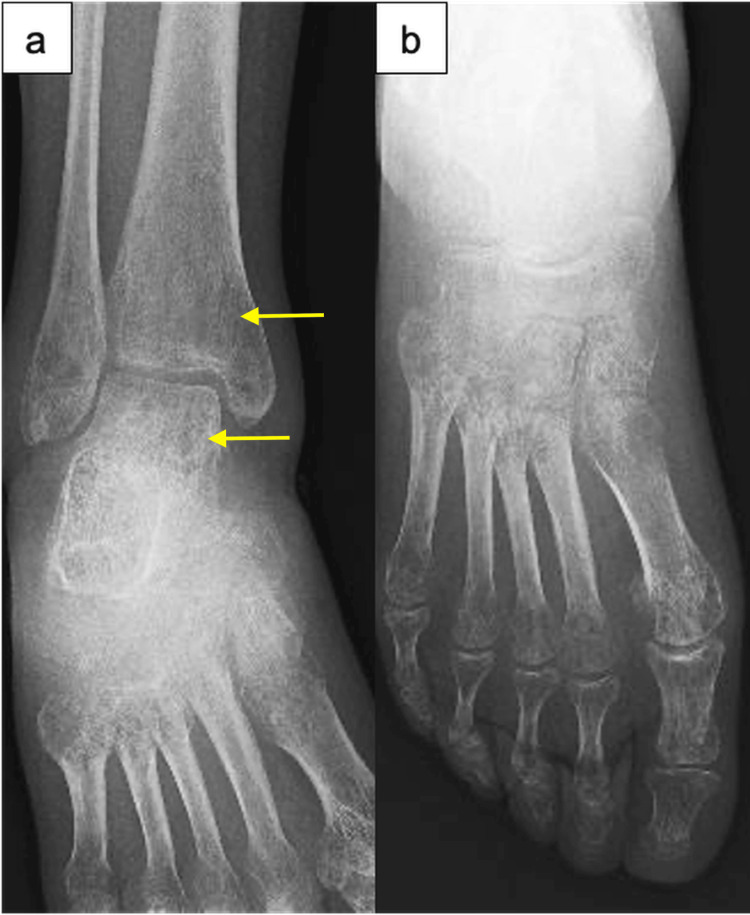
Serial anteroposterior radiographs of the right ankle and foot. At 1 week postpartum: (a) diffuse osteopenia in the distal tibia and fibula; (b) diffuse osteopenia in the calcaneus, navicular, cuboid, cuneiform, and phalanges. Arrows indicate representative areas of osteopenia in the distal tibia and talus.
